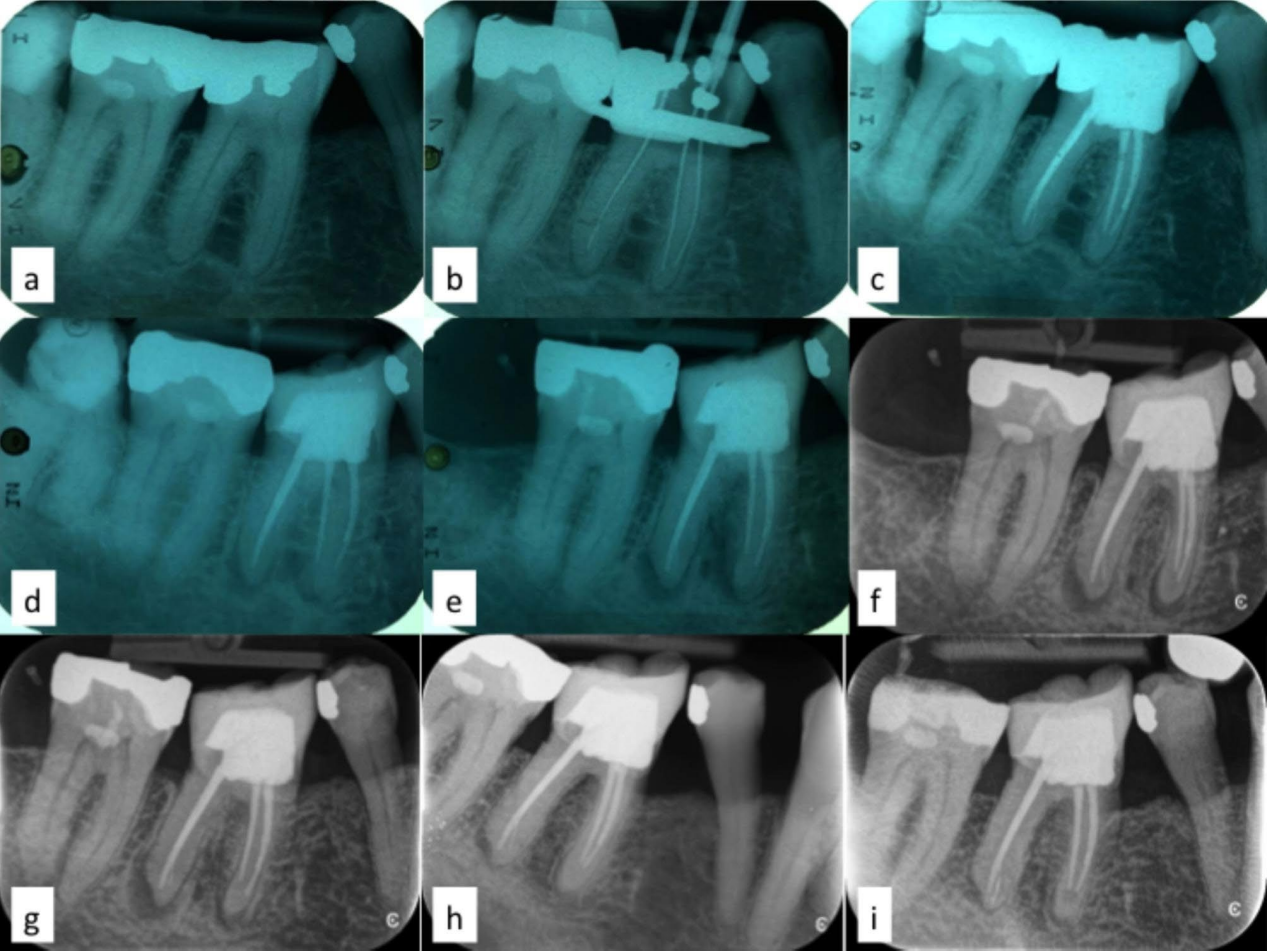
(a) Diagnostic periapical radiograph. (b) Working length radiograph. The tip of fractured instrument is detectable in the apical portion of the distal root canal. (c) Radiograph after root canal filling and core placing. (d) One year follow-up periapical radiograph showing lesions around the tips of the mesial and distal root. (e) Three years follow-up periapical radiograph showing increase of lesions. (f) Five years follow-up periapical radiograph with lesions at both root tips. Beginning of regular systemically lifelong prednisone intake. (g) Seven years follow-up periapical radiograph showing decrease of lesions even more pronounced at the mesial root. (h) Ten years follow-up periapical radiograph showing almost complete healing of the former lesions. (i) Eleven years follow-up periapical radiograph with nearly complete resolution of the former lesions.
